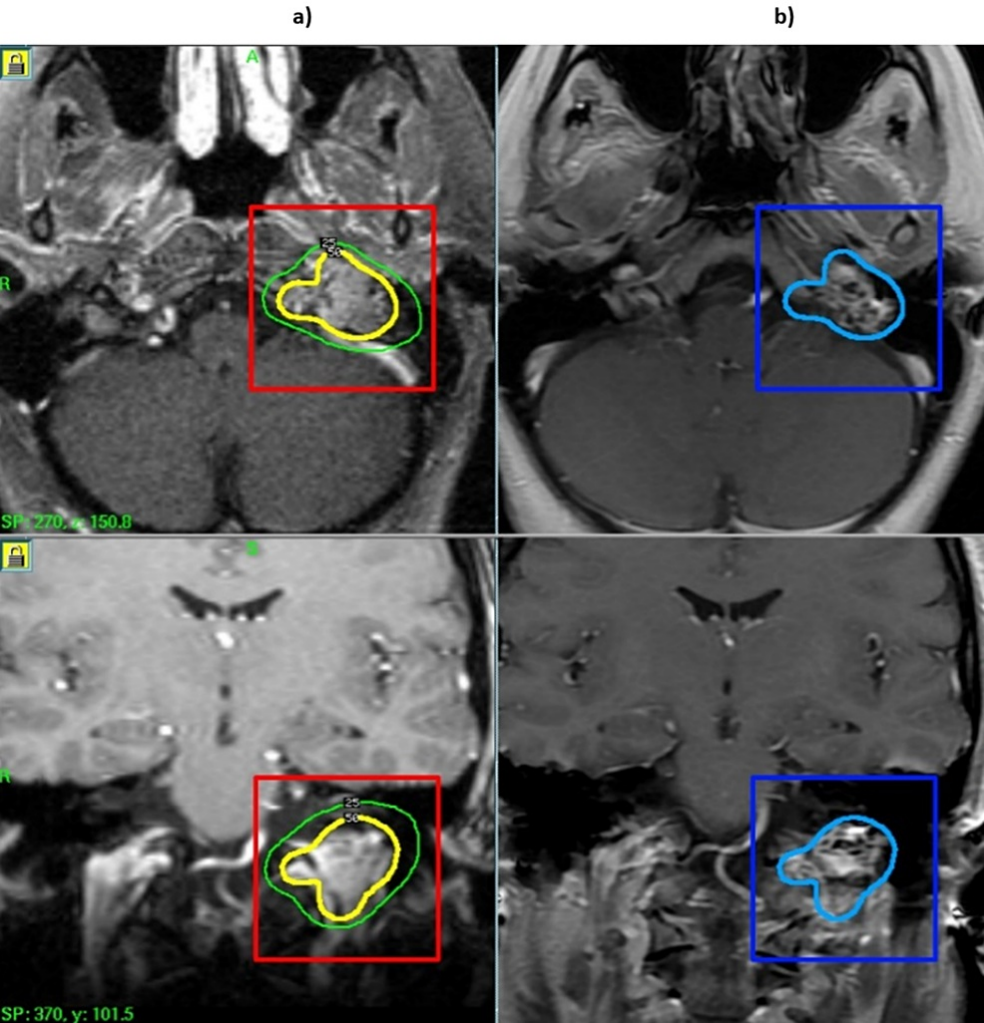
MRI of glomus jugulare tumor before and after GKRS (a) Pre-GKRS MRI of a patient with a 6.7-cc glomus tumor treated with 12 Gy to the 50% isodose line. (b) Post-GKRS MRI of the same patient five years after GKRS showing tumor shrinkage. GKRS, Gamma knife radiosurgery
